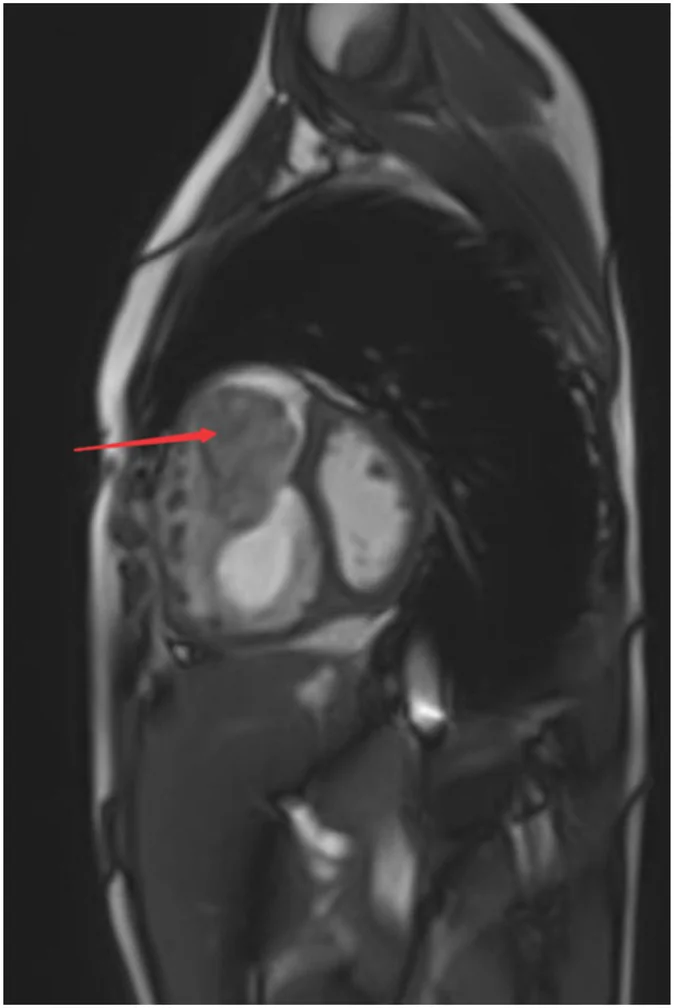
Sagittal view of cardiac magnetic resonance imaging showing RV mass (arrow).
